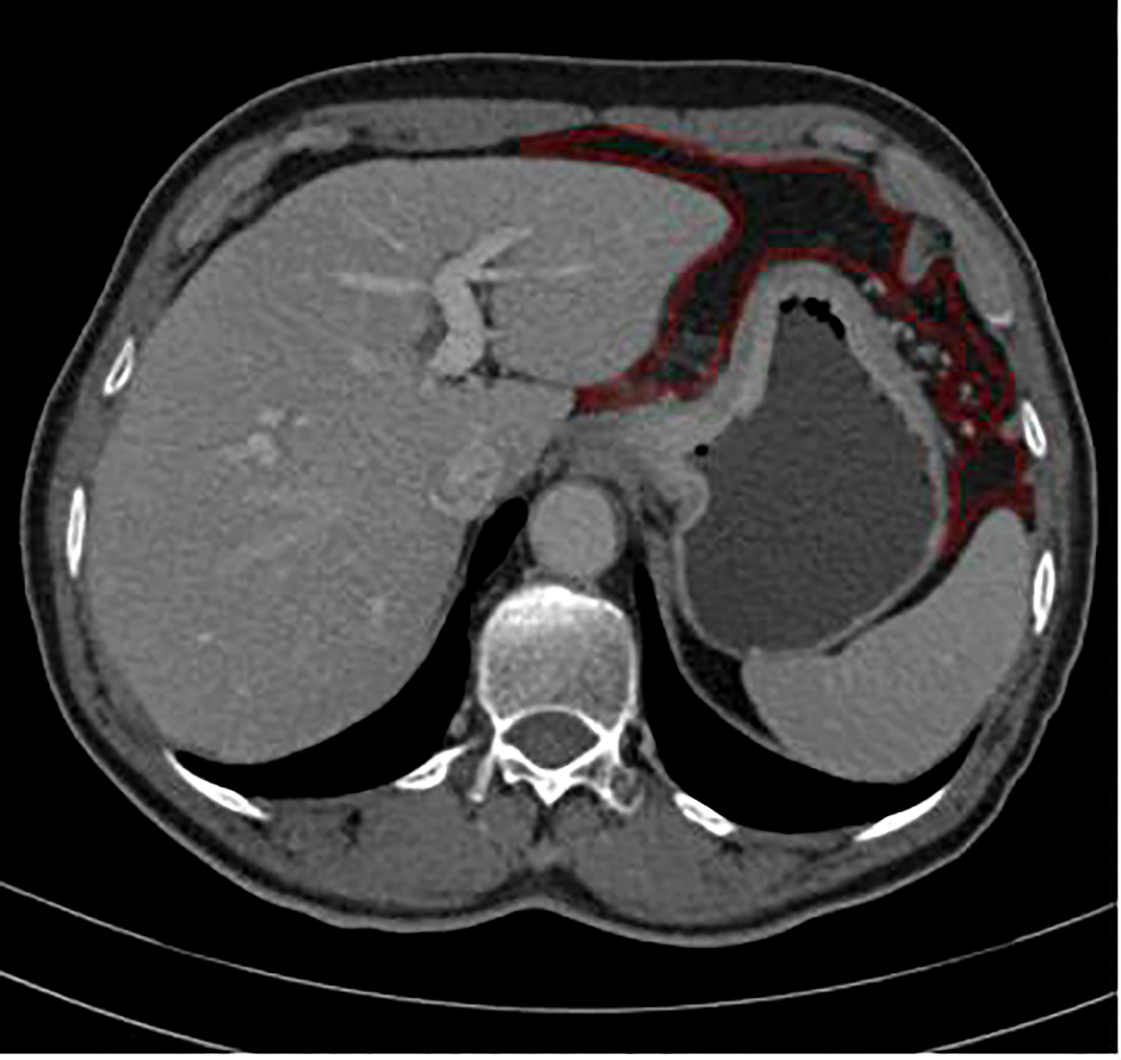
ROI annotation (red line) on a representative abdomen enhanced venous CT image, as an example of drawning ROI covering the peritoneotome or primary lesion slice by slice.
